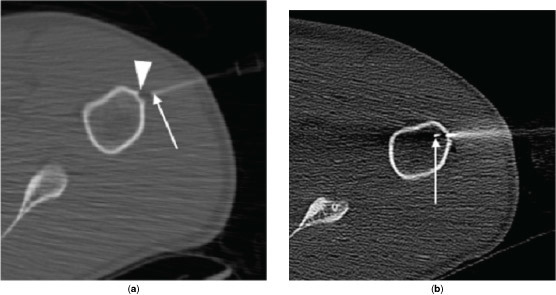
CT-guided core needle biopsy at initial presentation. (a) Axial CT scan, bone algorithm, of the left humerus during first pass biopsy showing location of Bard Truguide 17 G × 7 cm coaxial needle (Bard Biopsy Systems, Tempe, AZ) (arrow). Absence of cortical breech is observed (arrowhead). (b) Axial CT scan, bone algorithm, of the left humerus during second pass biopsy showing AprioMed Bonopty 15 G × 16 cm biopsy needle (Apriomed AB, Uppsala, Sweden) breaching the cortex with needle tip (arrow) located just within the medulla.
